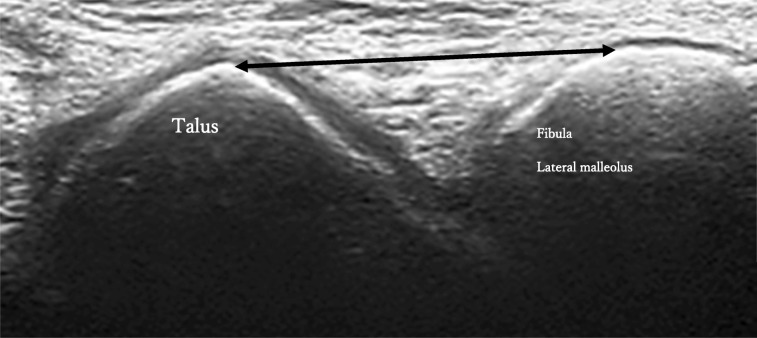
Ultrasound images of the fibular lateral malleolus and talus The probe is applied to the long axis of the fibular lateral malleolus and the anterior lateral portion of the talus as markers. We checked the anterior and posterior talus bone projections, which are drawn at sharp angles on the image. Subsequently, we checked the rounded fibular lateral malleolus and photographed them in B mode
